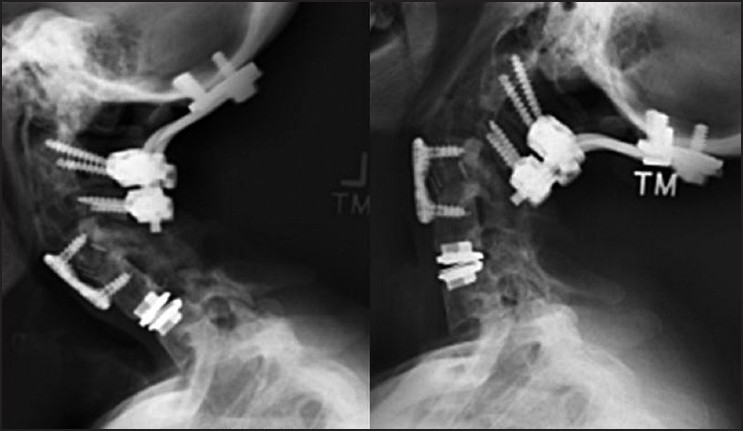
Final flexion/extension radiographs after prosthesis revision and anterior cervical fusion. Motion is retained at the level of the distal artificial disc, 1 year after implantation
